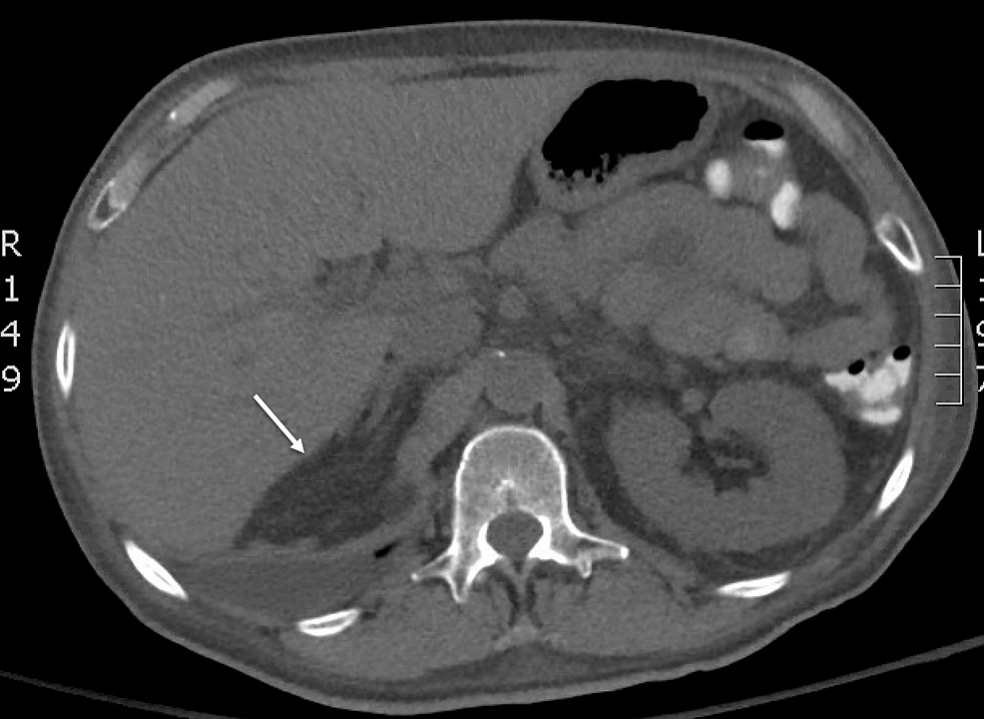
CT of the abdomen revealing malignant left hepatic lesion (arrow). CT: computed tomography
